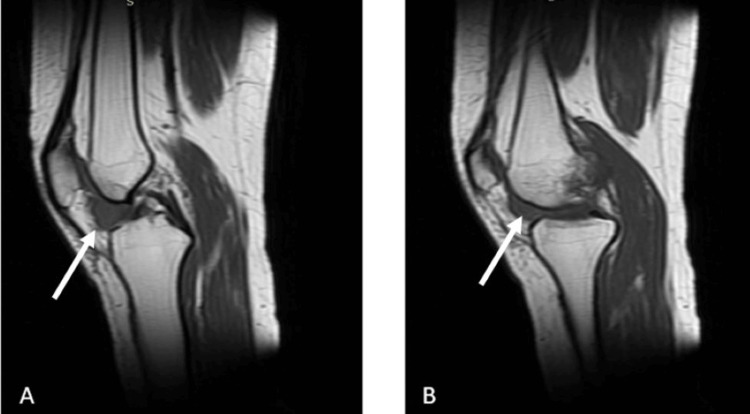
The patient's T1-weighted sagittal MRI sections. (A) The lesion appears as a hypointense area within the affected region, indicating its distinct contrast against the surrounding normal tissue. (B) Another sagittal section displaying the lesion’s extent and internal characteristics, further delineating its borders and involvement The white arrows indicate the lesion MRI: magnetic resonance imaging
